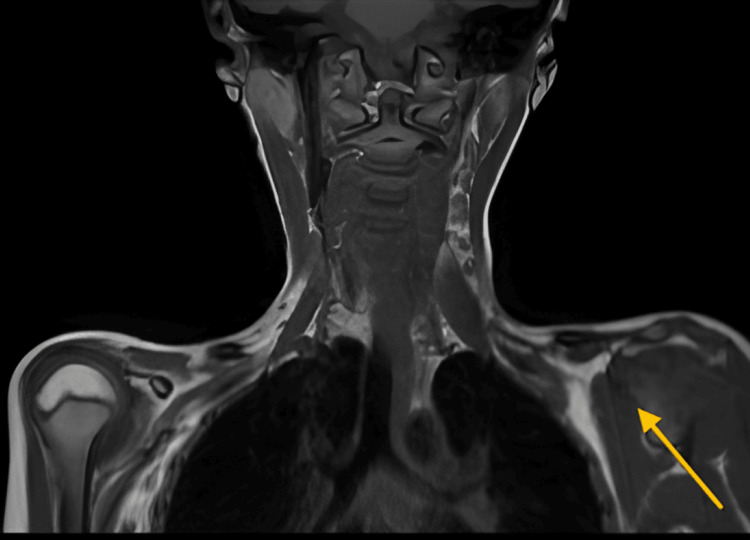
MRI brachial plexus of a seven-year-old girl, suggesting right preganglionic injury (yellow arrow) MRI: magnetic resonance imaging
